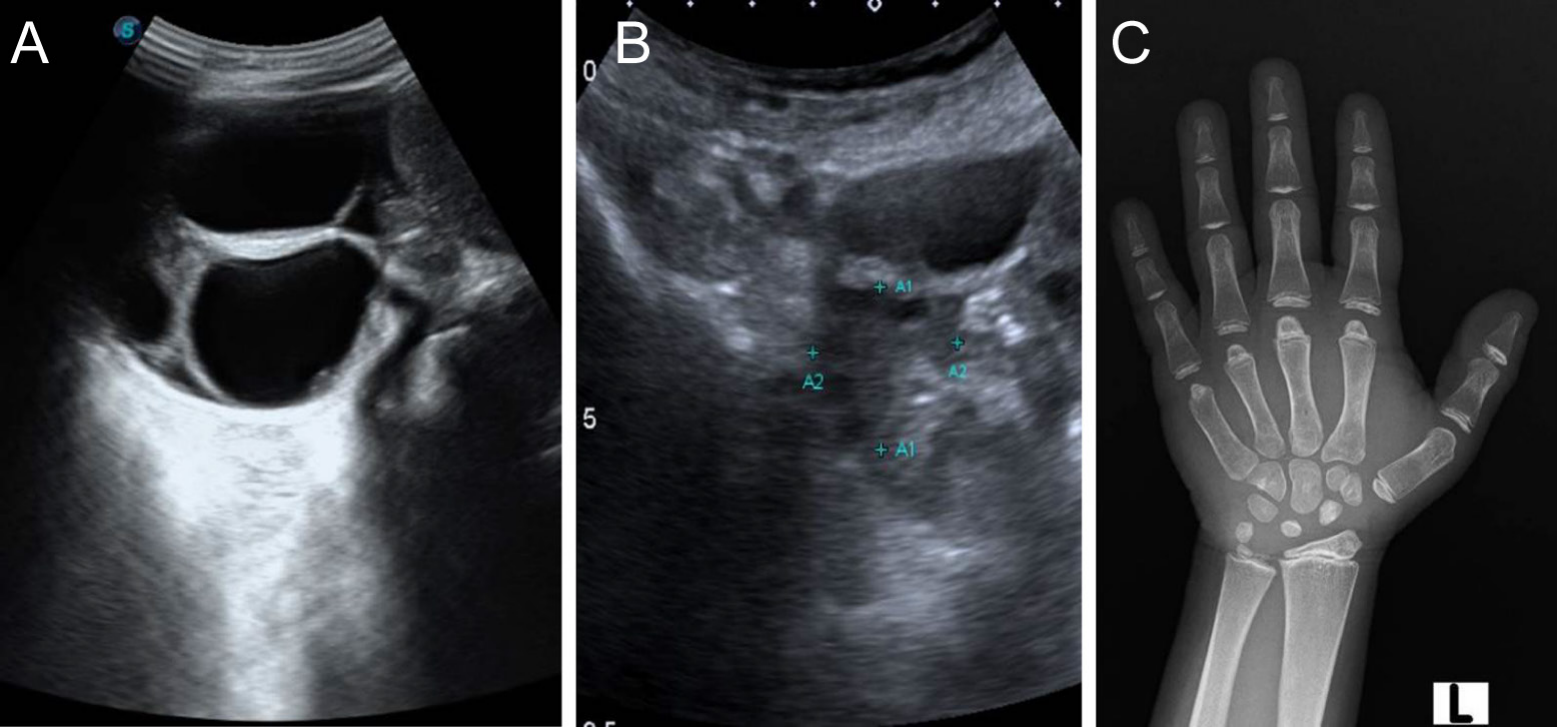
(A) Ultrasound of the abdomen at presentation with multiple cysts and ovarian volume of 50 mL. (B) Ultrasound abdomen at follow-up with resolved cyst. (C) P1 presented at 21 years with a delayed bone age of 9 years.
